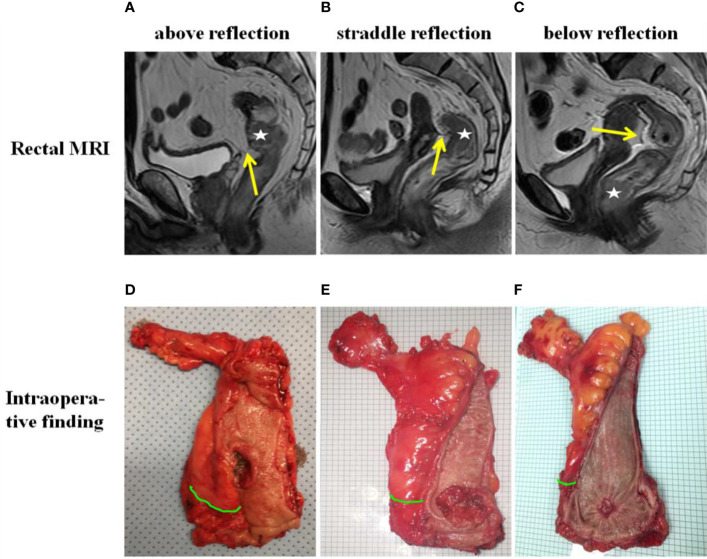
Tumor location relative to the anterior peritoneal reflection (APR) as determined by MRI (A–C) and intraoperative palpation and visualization (D–F). The “☆” in the MRI indicates the tumor. The yellow arrow in the MRI indicates the APR. The green curve in intraoperative finding indicates the APR.
